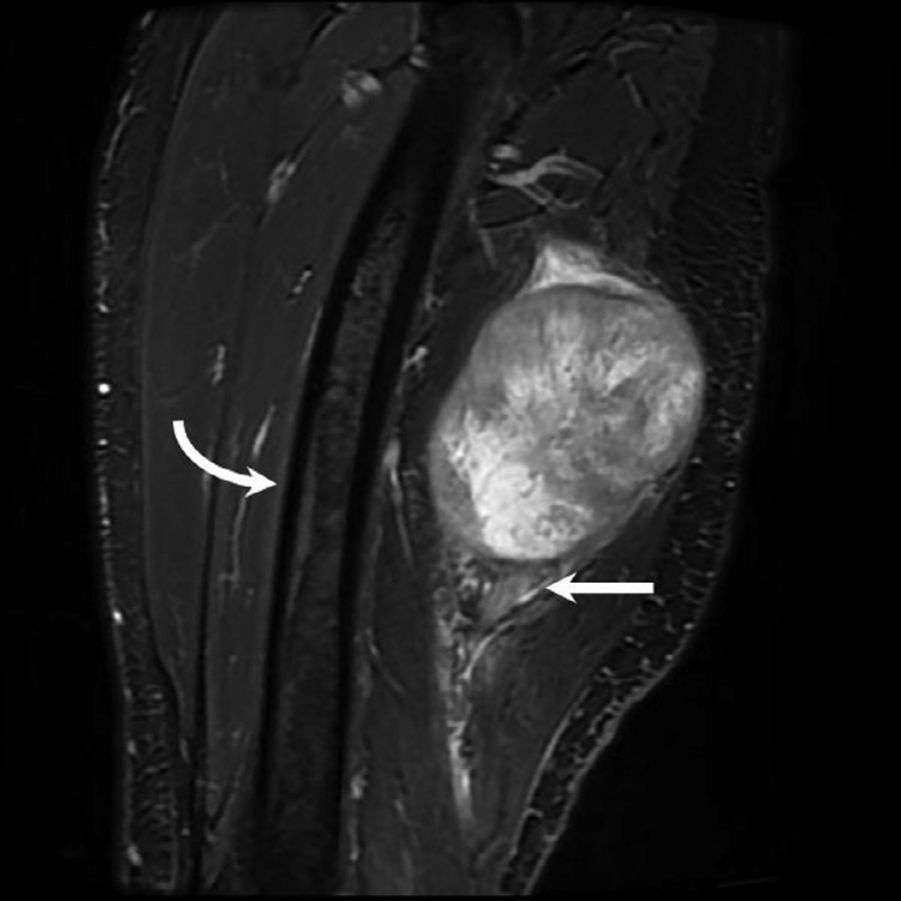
Sagittal T2 fat-saturated MRI image shows the mass is contiguous with the sciatic nerve distally (arrow). There is no osseous involvement of the femur which is anterior to the mass (curved arrow).
